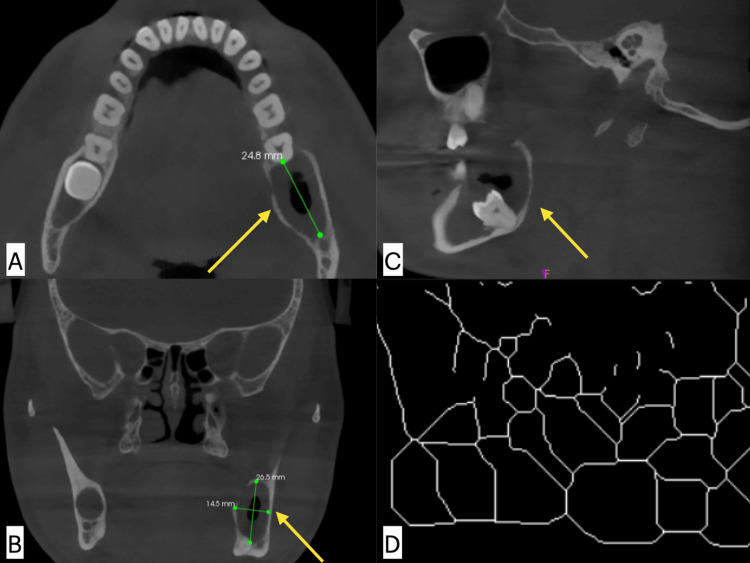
Dentigerous cyst (DC) in CBCT images measurement of the size of the lesion in (A) axial, (B) coronal, (C) sagittal planes, and (D) skeletonised fractal image. CBCT: cone beam computed tomography
